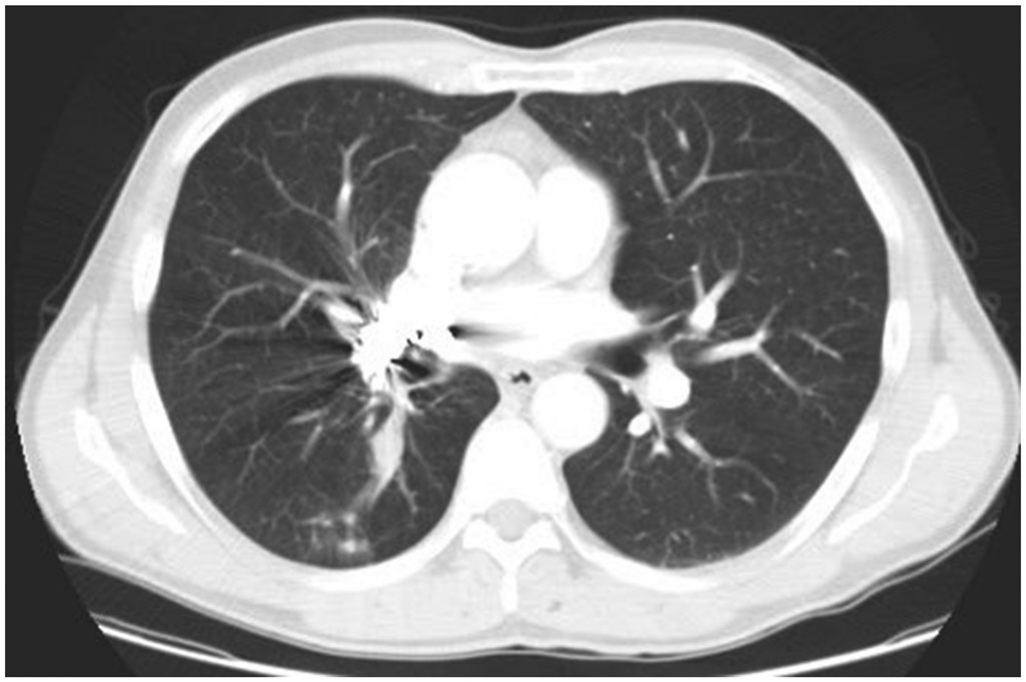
Thoracic CT scan image in 2009. A follow-up contrast-enhanced CT image with soft-tissue window after 2 months of treatment with second-line chemotherapy showing an increased tumour in the right lung.
